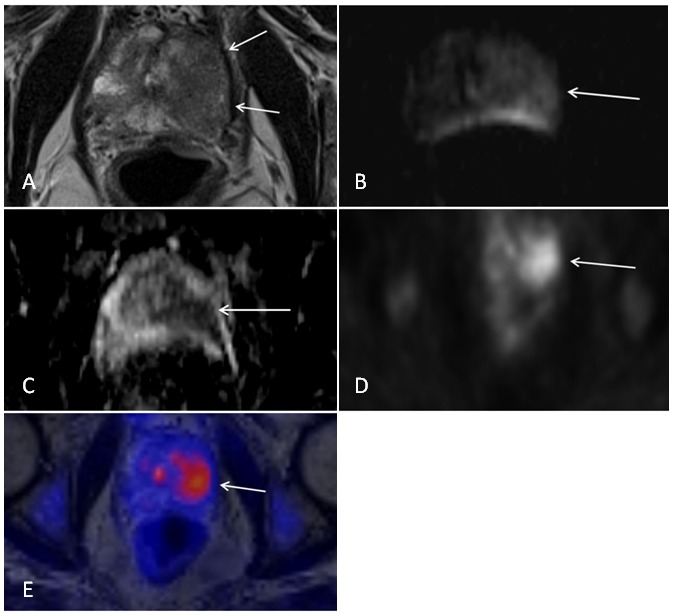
Patient with a biopsy proven prostate cancer (Gleason score 3+4 = 7) of the left peripheral and transitional zone. Images from simultaneous [^18^F] choline PET/MRI. A) T2-weighted image displaying a large hypo-intense lesion of the left peripheral and transitional zone. B) Diffusion-weighted image (b = 1000) showing a hyper-intense signal of the lesion. C) Corresponding ADC map with a hypo-intense delineation of the lesion. D) PET image indicating of focal choline uptake of the lesion. E) Fused MRI/PET image.
